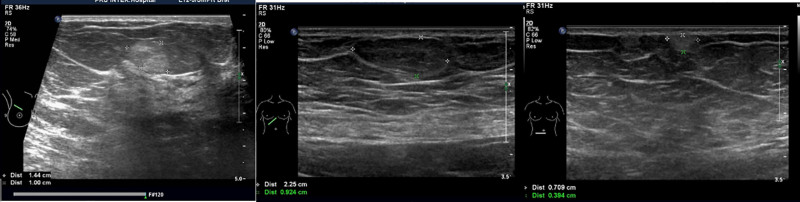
Ultrasound examination showed masses of other parts of the body.
